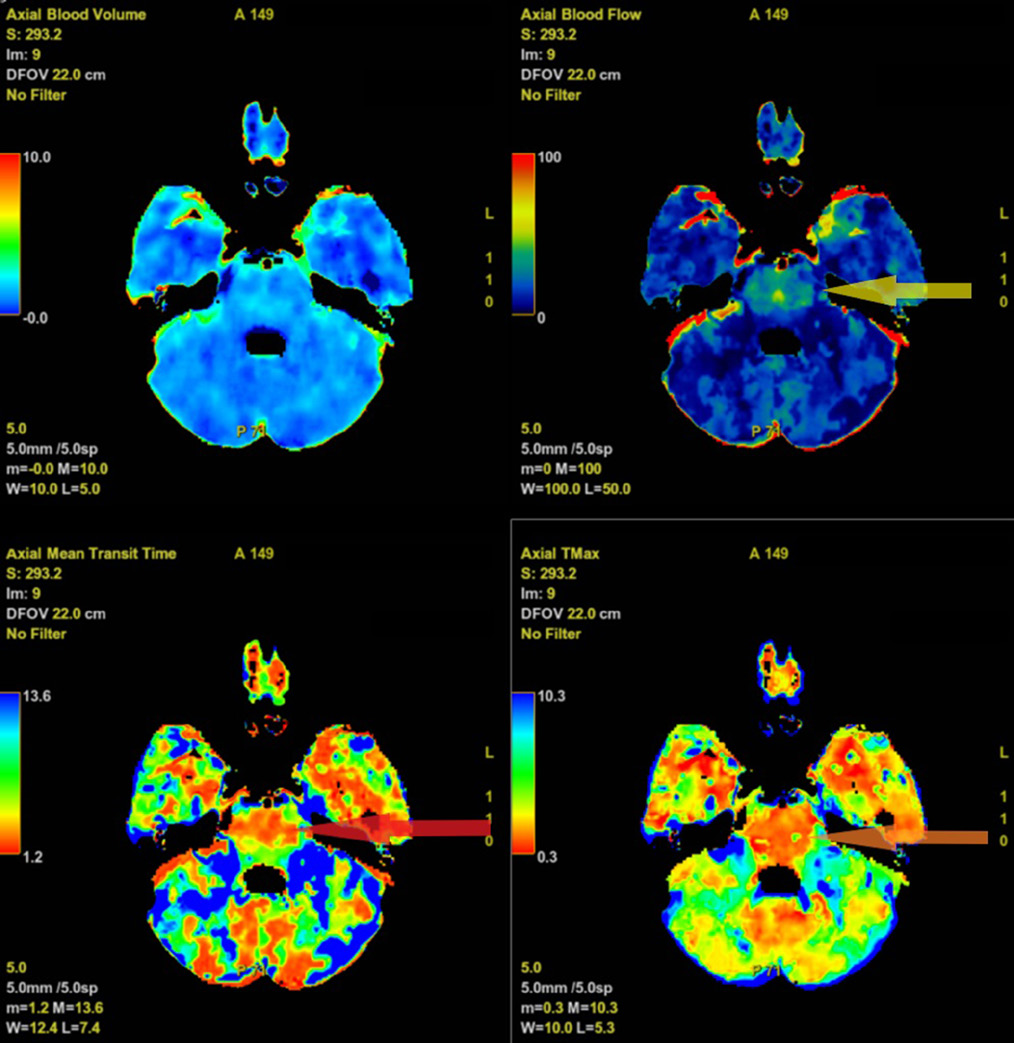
CT perfusion images at the level of the pons. This demonstrates increased blood flow (top right, yellow arrow), reduced mean transit time (bottom left, red arrow) and reduced time-to-maximum (bottom right, orange arrow) in the upper pons.
